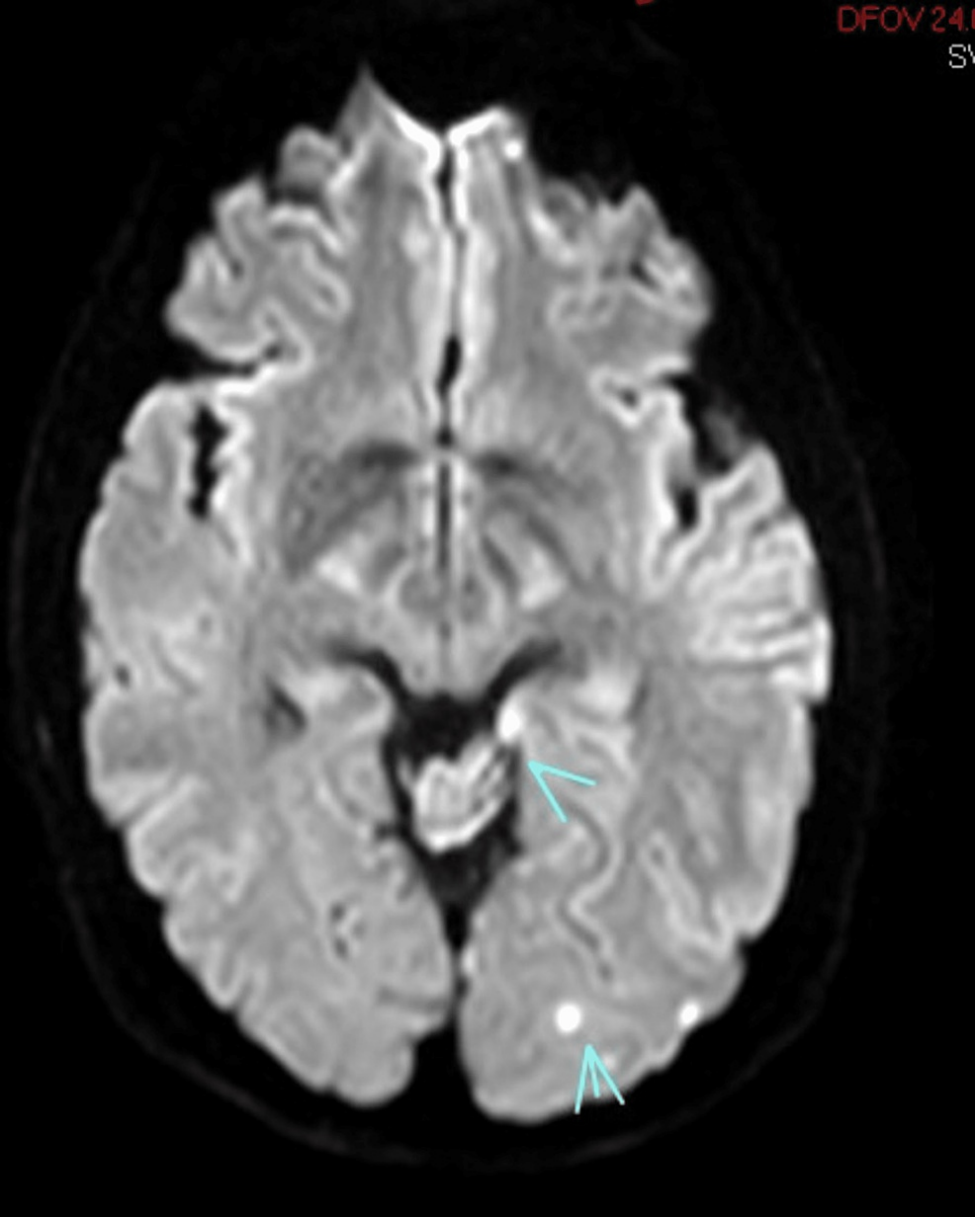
MRI of the brain demonstrating foci of acute/early subacute ischemic infarction in the left PCA distribution, involving the left occipital cortex and the posterior body to tail of the hippocampus PCA: posterior cerebral artery
